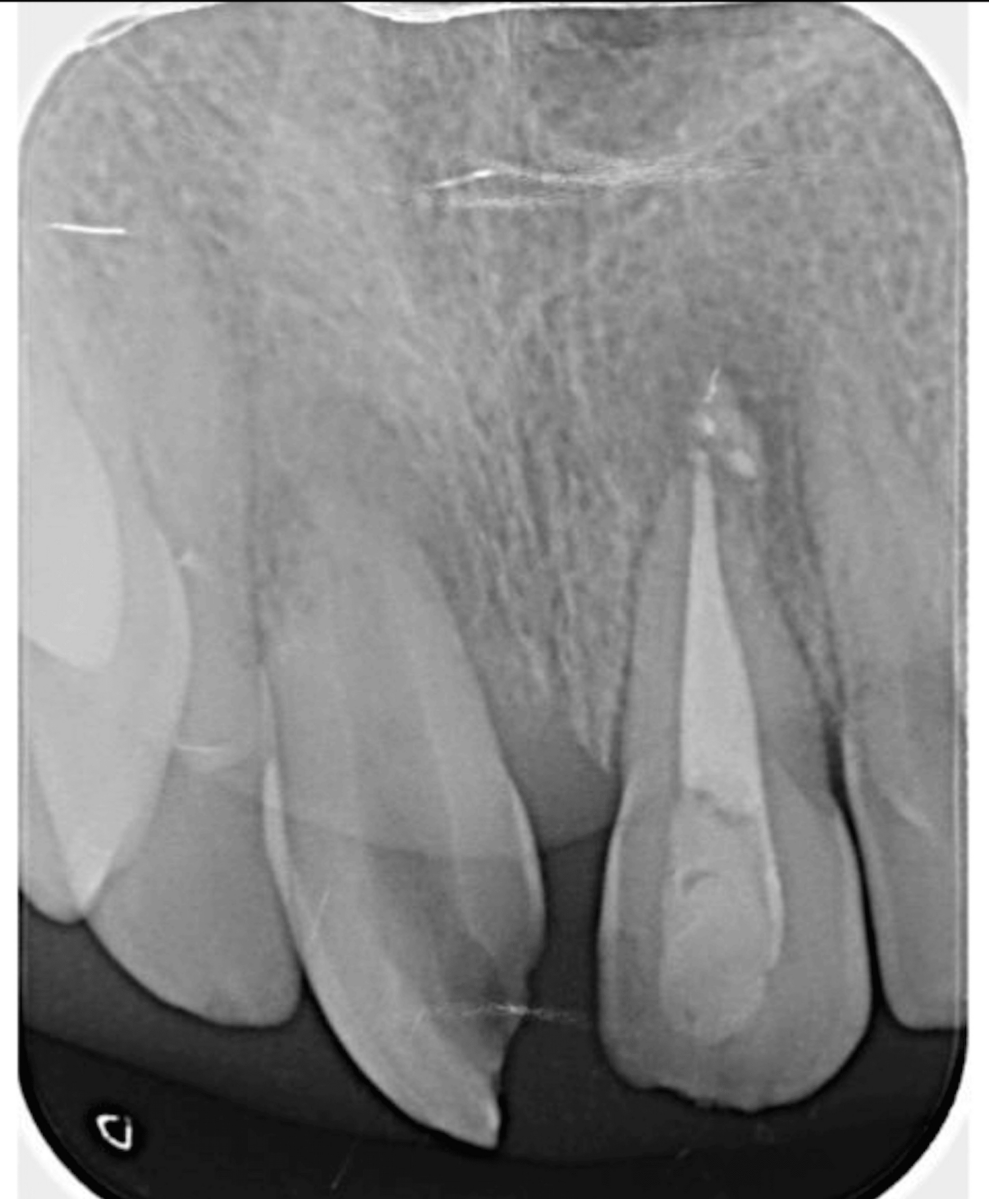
Postoperative radiograph.
